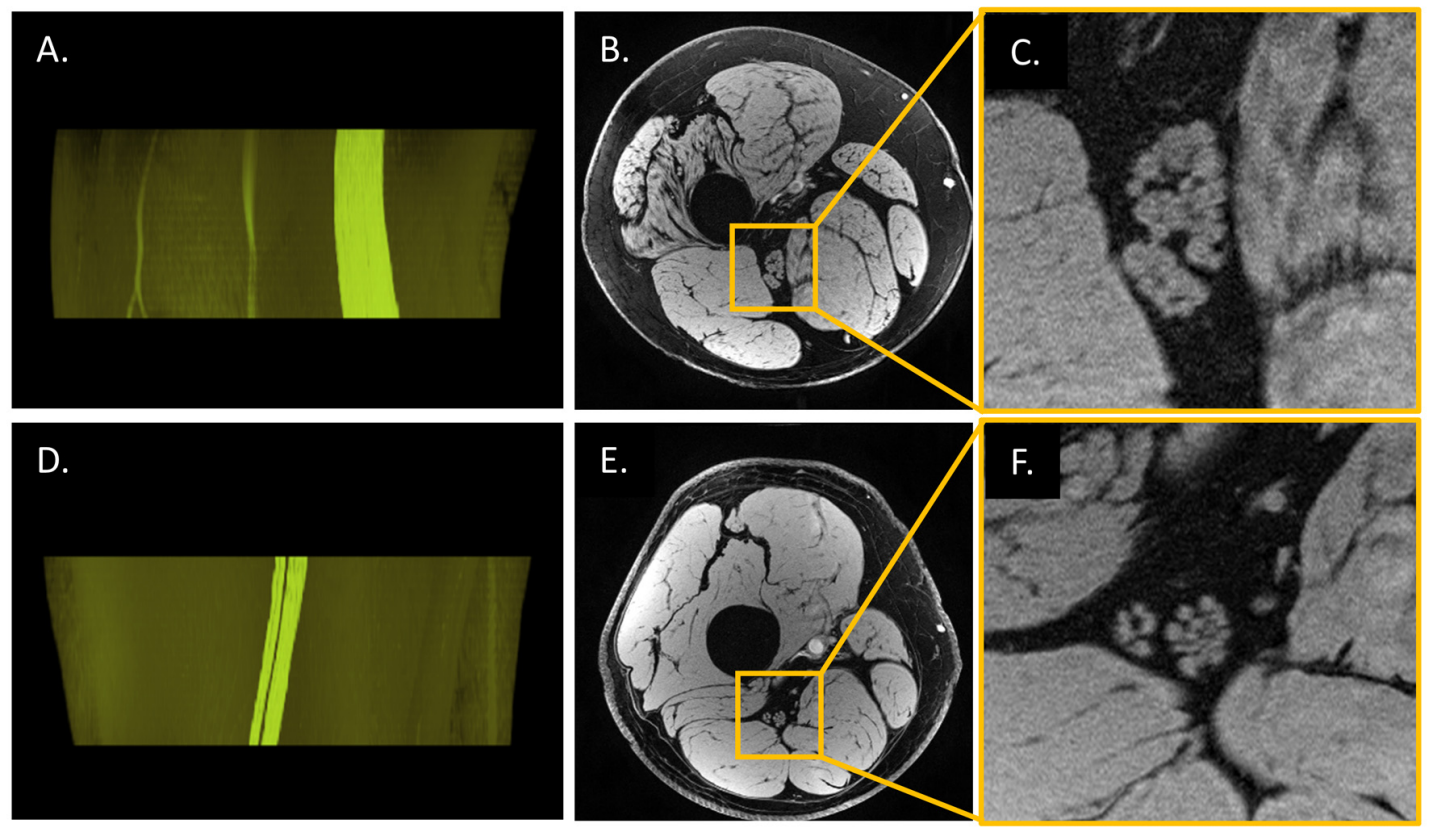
In vivo* ultra-high-resolution magnetic resonance imaging of sciatic nerve in a patient with Charcot–Marie–Tooth disease (CMT) and healthy control. Images of a patient with CMT type 4J (
